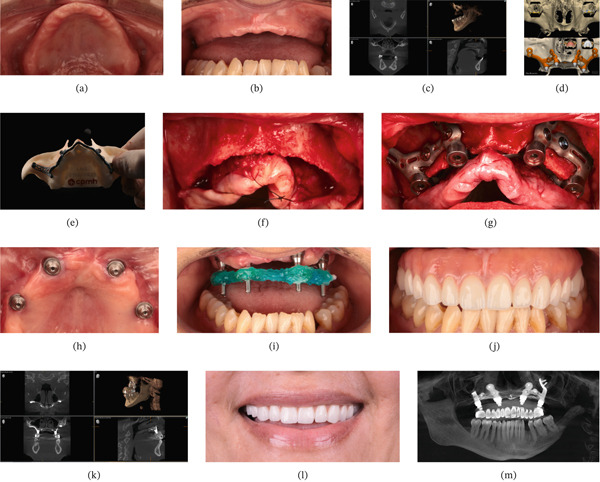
Clinical presentation of Case 1. (a) Occlusal view of Patient 1. (b) Intraoral frontal view of the initial situation of Patient 1. (c) The CBCT scan of Patient 1 revealed an important lack of alveolar bone in all maxillary regions. (d) Virtual planning of the subperiosteal implant and three‐dimensional reconstructions of the subperiosteal implant design for the upper jaw. (e) The fabricated CSI model was screwed to a printed skull for evaluation. (f) Elevation of the mucoperiosteal flap. (g) Subperiosteal implant placed in Patient 1. (h) After 4 months of healing, the temporary immediate restorations were removed. (i) The splinted impression technique was used to accurately transfer the implant position from the mouth to the definitive cast. (j) Intraoral frontal view of the final delivery of the prosthesis. (K) Baseline control radiography. (l) Intraoral view of the final prosthesis after 42 months of follow‐up. (m) Panoramic radiography image showing the status of the implant.
